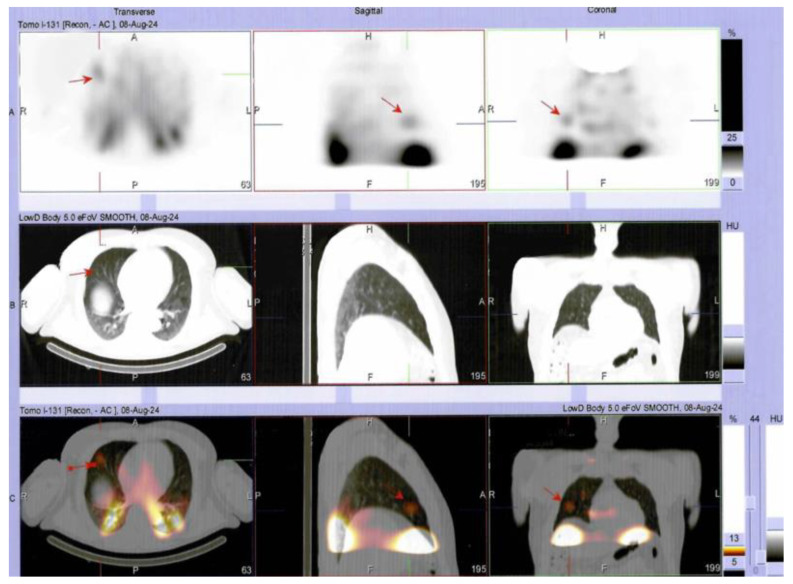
Additionally, SPECT/CT findings revealed areas with moderate uptake in the right pulmonary parenchyma, suggesting lung metastases (upper row (A): SPECT image; middle row (B): CT image, lower row (C): SPECT/CT fusion image).
